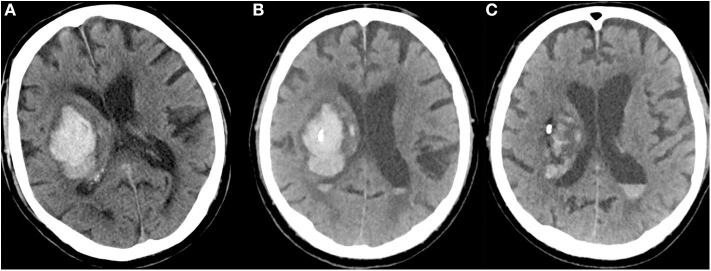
(A) CT of a 79-year-old man with a right-sided, VKA-related deep ICH at admission (NIHSS = 14; INR = 3.0; hematoma volume: 58 ml). (B) CT after bedside catheter placement and aspiration of 11 ml of blood shows that the catheter tip is well positioned in the core of the hematoma (after administration of 4000 PCC, INR = 1.2). (C) After application of urokinase (5000 IE every 6 h) for four consecutive days, the hematoma volume had decreased to 21 ml (NIHSS = 10).
